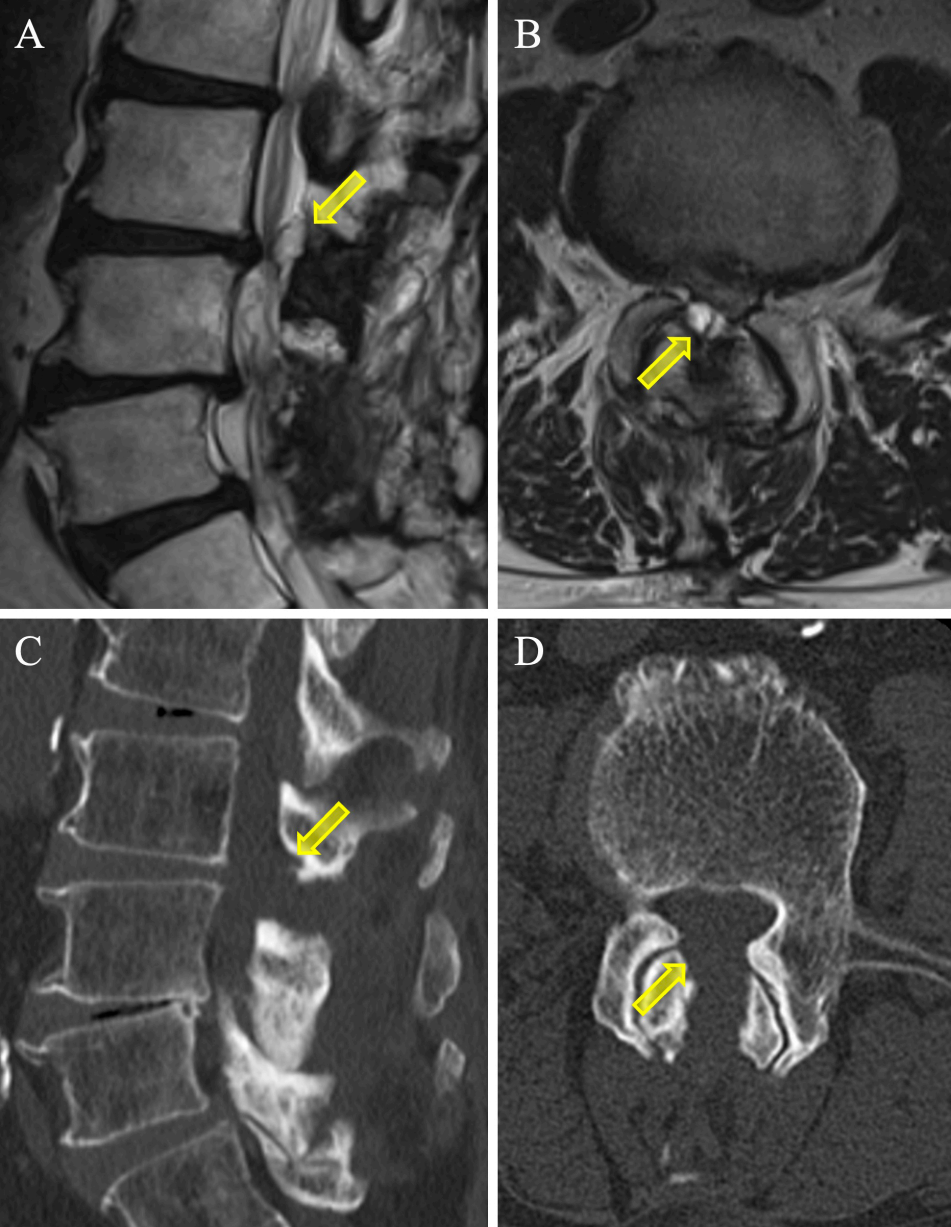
Preoperative T2-weighted MRI images (A, sagittal; B, axial) and CT scans (C, sagittal; D, axial) showing the dural sac severely compressed by a synovial cyst from the right facet at the L3/4 level (*arrow,* synovial cyst).
